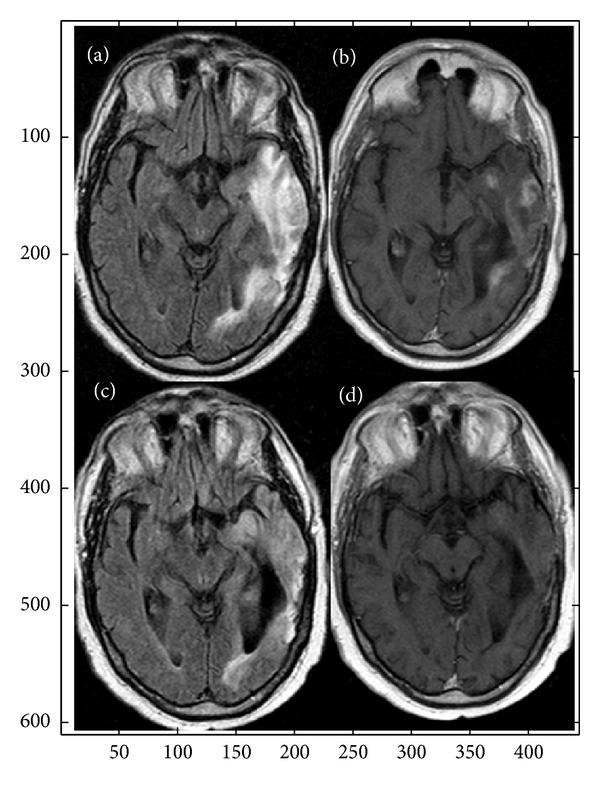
Before treatment MRI SE FLAIR (a) and T1 after contrast medium infusion (b) shows a large lesion in the left temporal lobe, hyperintense on FLAIR image with multiple focal enhancement areas after c.m. infusion. There is a compression on ventricle trigone. MRI FLAIR (c) and T1 (d) after contrast medium (c.m.), after treatment, show marked reduction of the hyperintensity area on the FLAIR sequence with disappearance of the enhancement areas on T1 sequence after c.m. The ventricle trigone is enlarged.
